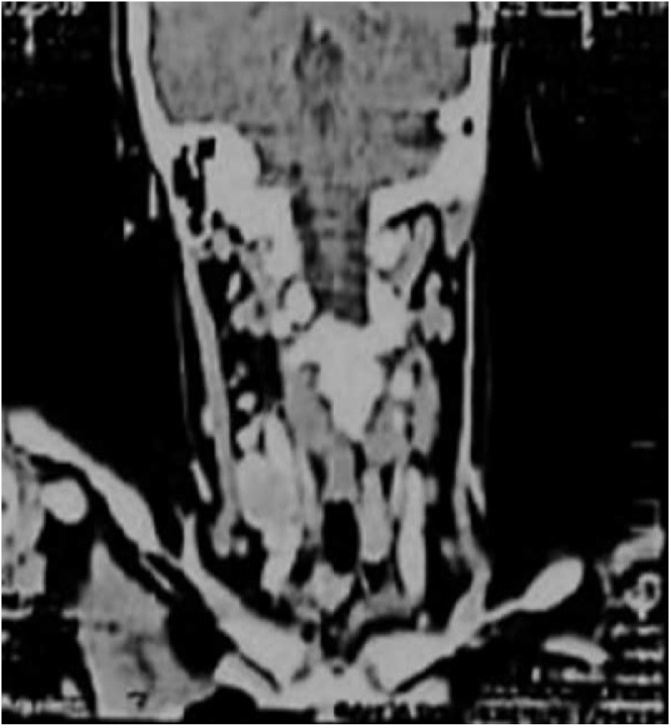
Post right thyroidectomy and isthmectomy CT neck coronal enhanced view showing metastatic nodes on right side at level IV and level VI compressing the internal jugular vein.
